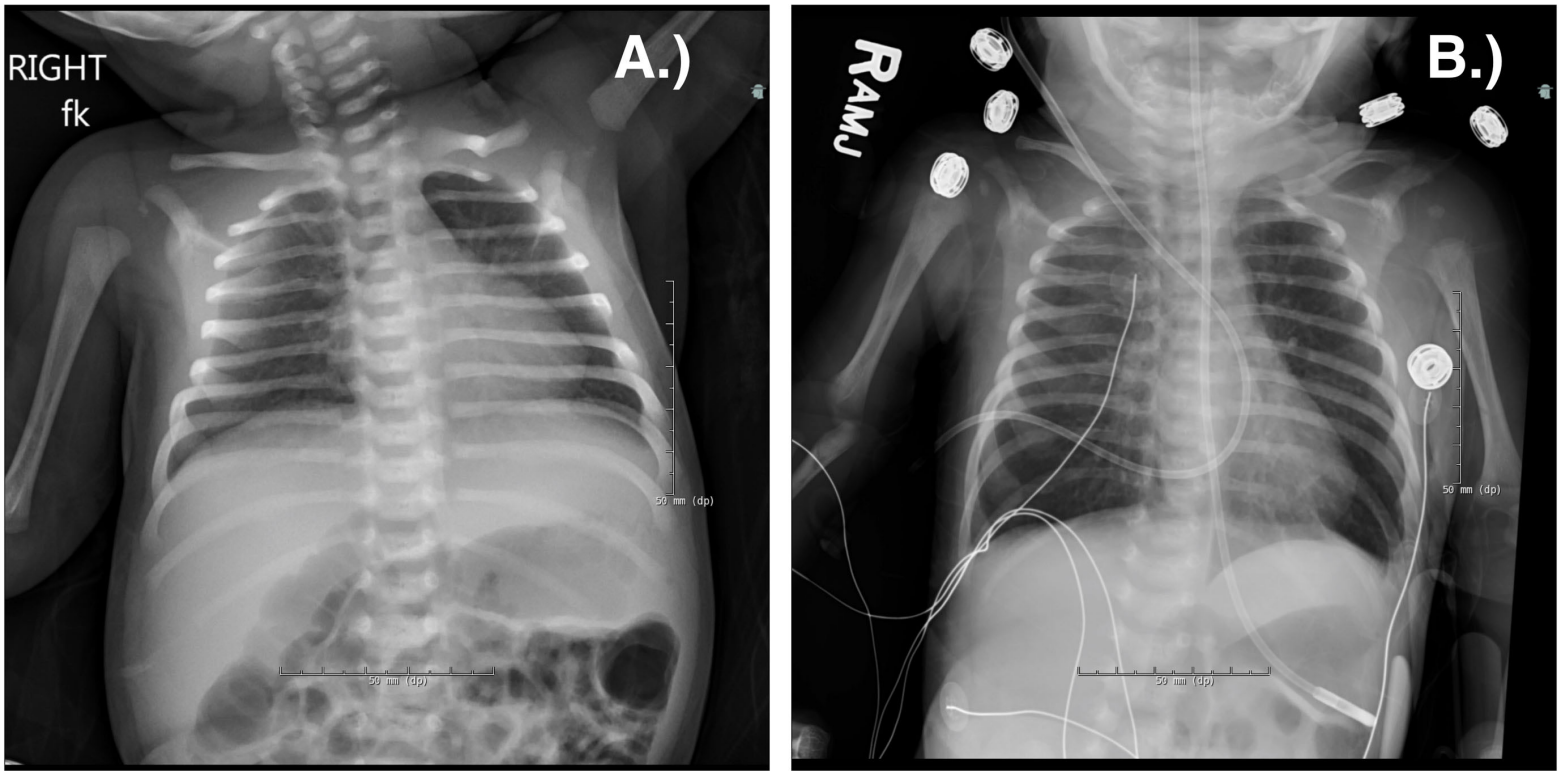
Chest radiograph of a term male infant with X-linked severe combined immune deficiency and RSV pneumonitis, which was rapidly fatal despite adjunctive use of IVIG and inhaled ribavirin. He was treated with an infusion of maternal haplo-identical hematopoietic stem cells at 18 days of life. He was admitted at 10 days old (A) and died at 27 days old (B) due to worsening respiratory status. Note the absent thymus.
